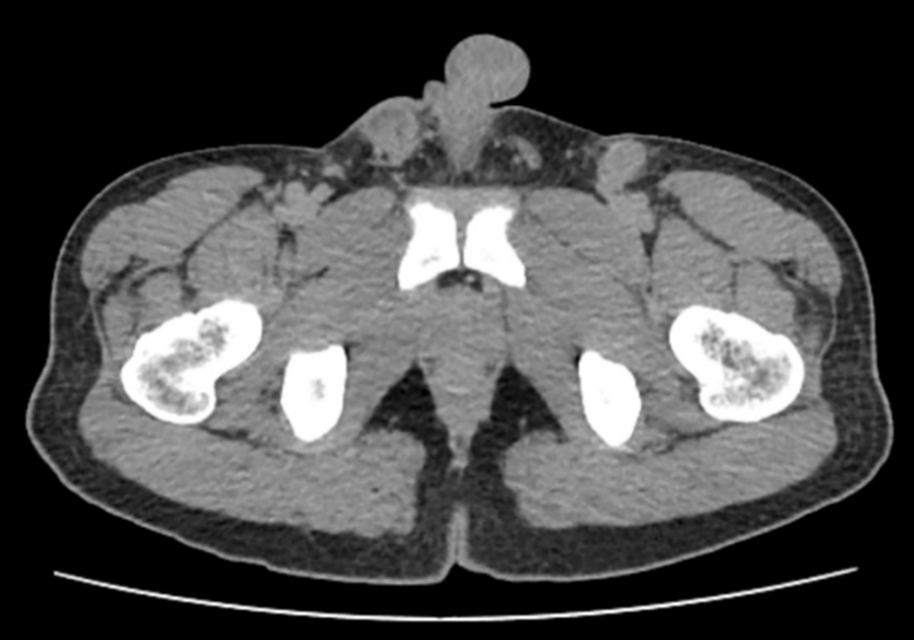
Contrast-enhanced computed tomography (CECT) pelvis in axial view showed enlarged iliac lymph node and inguinal lymph nodes with necrotic center.
